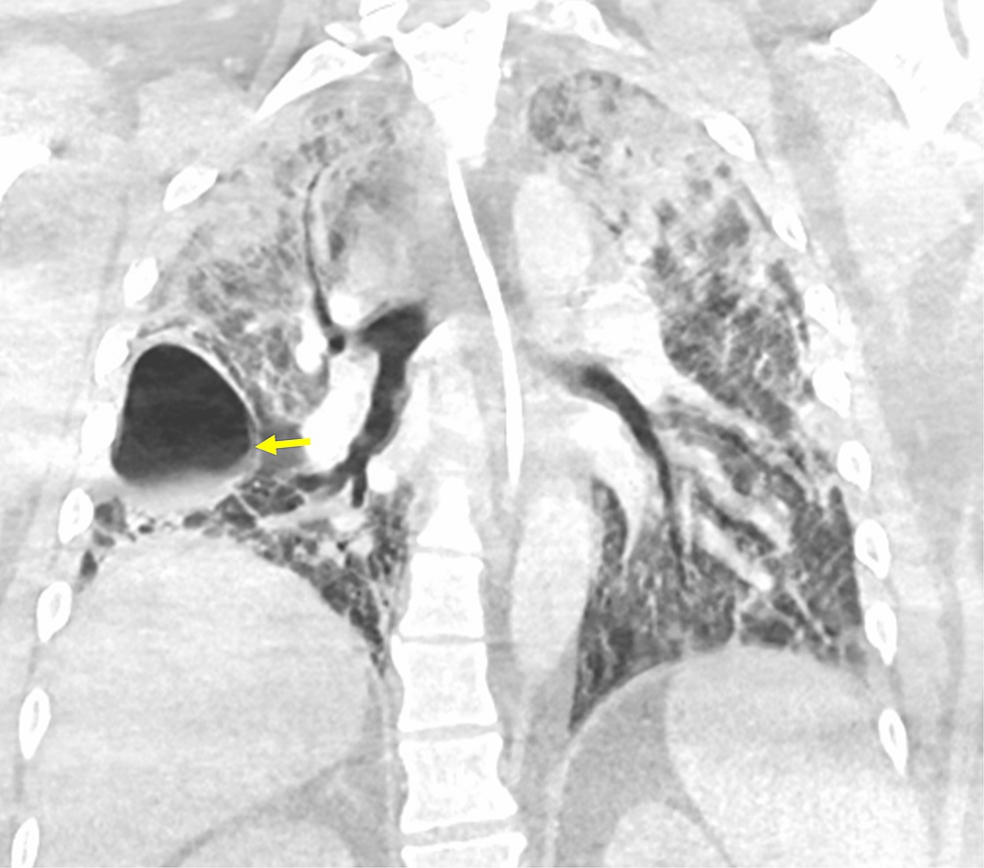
CT scan of the chest with contrast (coronal image) shows bronchiectasis involving predominantly lower lobes, reticular interstitial opacities, pneumatocele (yellow arrow), and upper lobe airspace opacities
